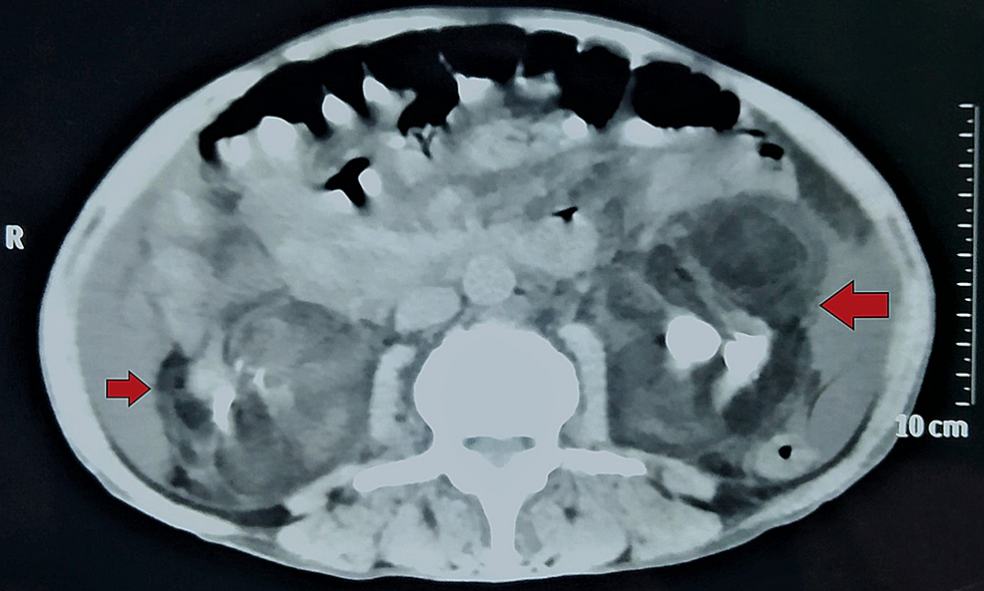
Non-contrast computed tomographic scan of the abdomen (axial section) demonstrating bilateral renal parenchymal replacement with fatty tissue (red arrow) and calculi in the pelvis.
